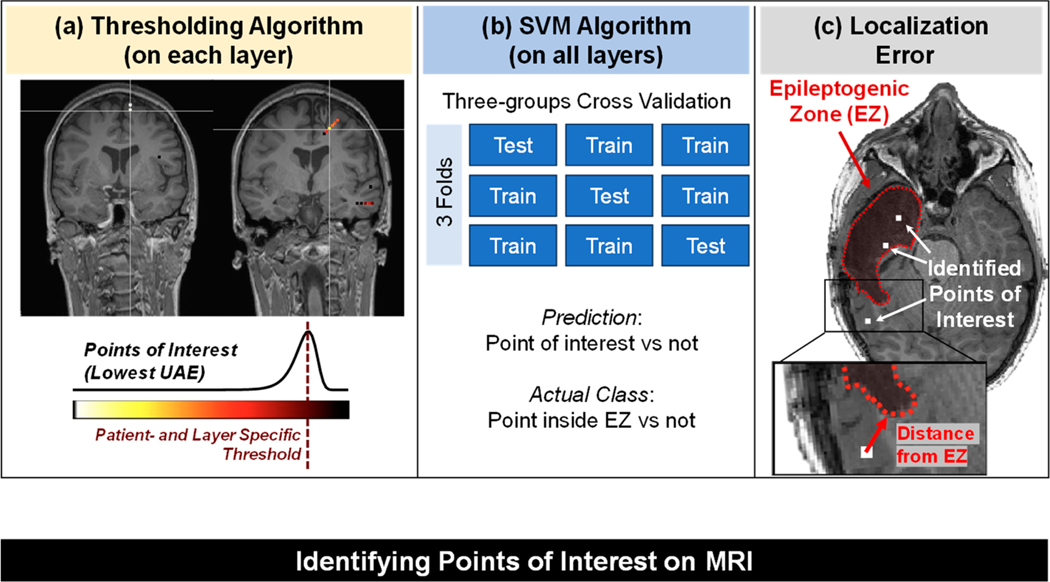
Identifying points of interest (POIs) on the patient’s MRI.
